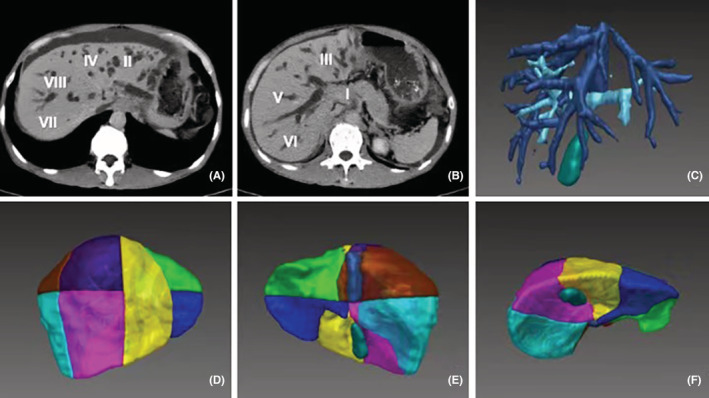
Assessment of drainage volume using 3D modeling. (A,B) Contrast‐enhanced CT scan of MHBO patient. (C) Reconstruction of the hepatic vein and portal vein system. (D,E,F) Ventral, dorsal, and caudal sight of 3D hepatic segments.
